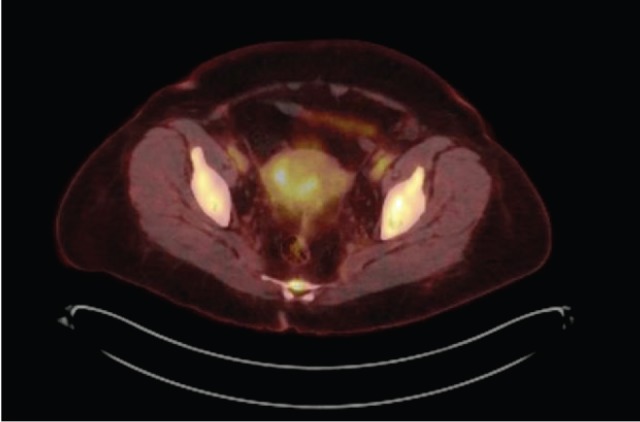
Increased FDG uptake on PET CT including uptake in uterus. PET CT scan. 1. Interval increase in FDG uptake of multiple hypermetabolic osseous metastases throughout the axial and proximal appendicular skeleton. 2. Mildly increased FDG uptake of mildly hypermetabolic mediastinal lymph nodes. 3. Moderate FDG uptake of the uterus centrally that was not present on imaging performed ten months prior.
